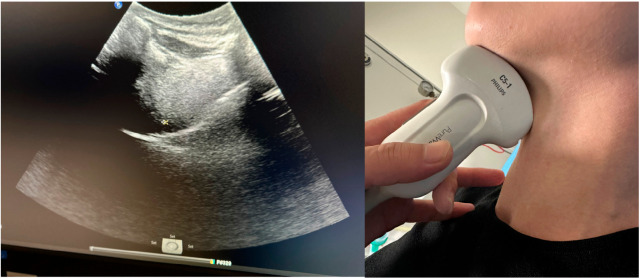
Ultrasonographic measurement of tongue thickness (TT): convex probe placed submentally (right) and the corresponding sonographic view (left).
